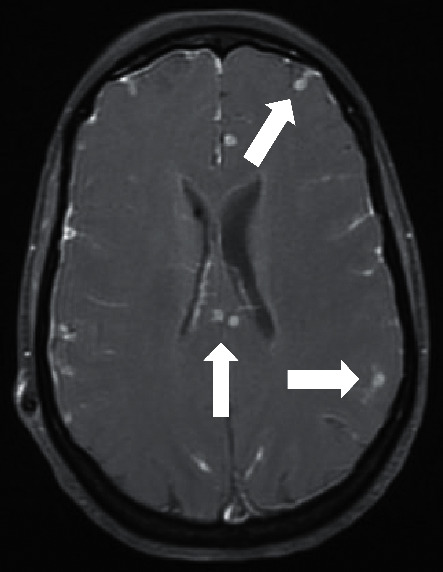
Brain MRI: T1, after gadolinium administration; arrows: multiple cryptococcoma.
